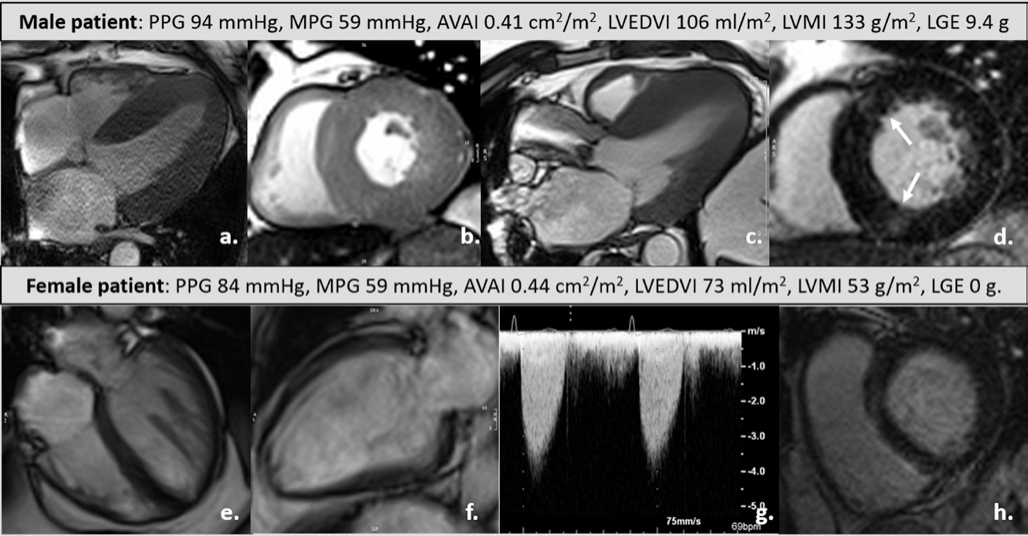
An example of a male (top row) and female (bottom row) patient with similar degree of aortic stenosis, showing cine stills (a-c, e, f), LGE imaging (d, h) and Continuous Wave Doppler through the aortic valve on echocardiography (g). AVAI, aortic valve area index (to BSA); LGE, late-gadolinium enhancement; LVEDVI, left ventricle end-diastolic volume index; LVMI, left ventricle mass index; MPG, mean pressure gradient; PPG, peak pressure gradient.
